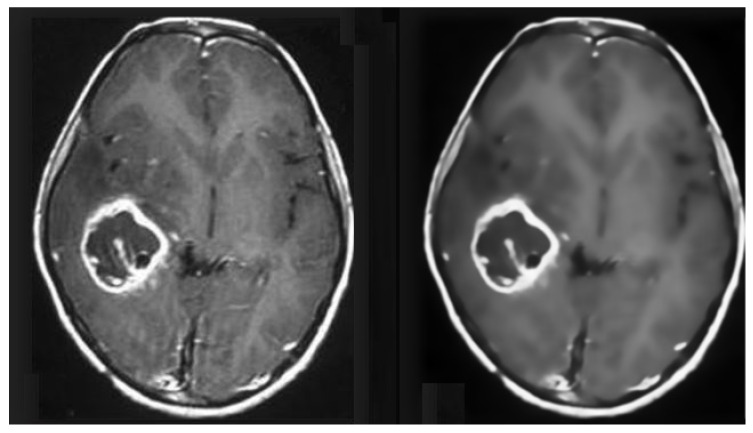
Pre-processed MRI data (Before and After Denoising).
